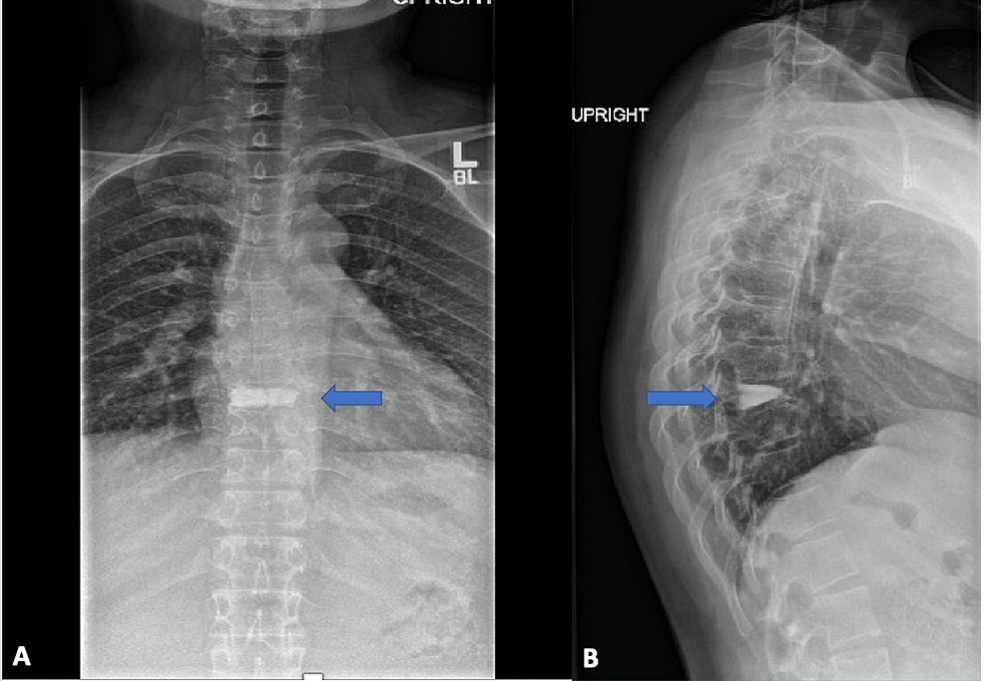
X-ray thoracic spine anteroposterior (AP) and lateral view A, X-ray thoracic spine AP view showing T9 kyphoplasty (blue arrow); B, X-ray thoracic spine lateral view showing T9 kyphoplasty (blue arrow)
